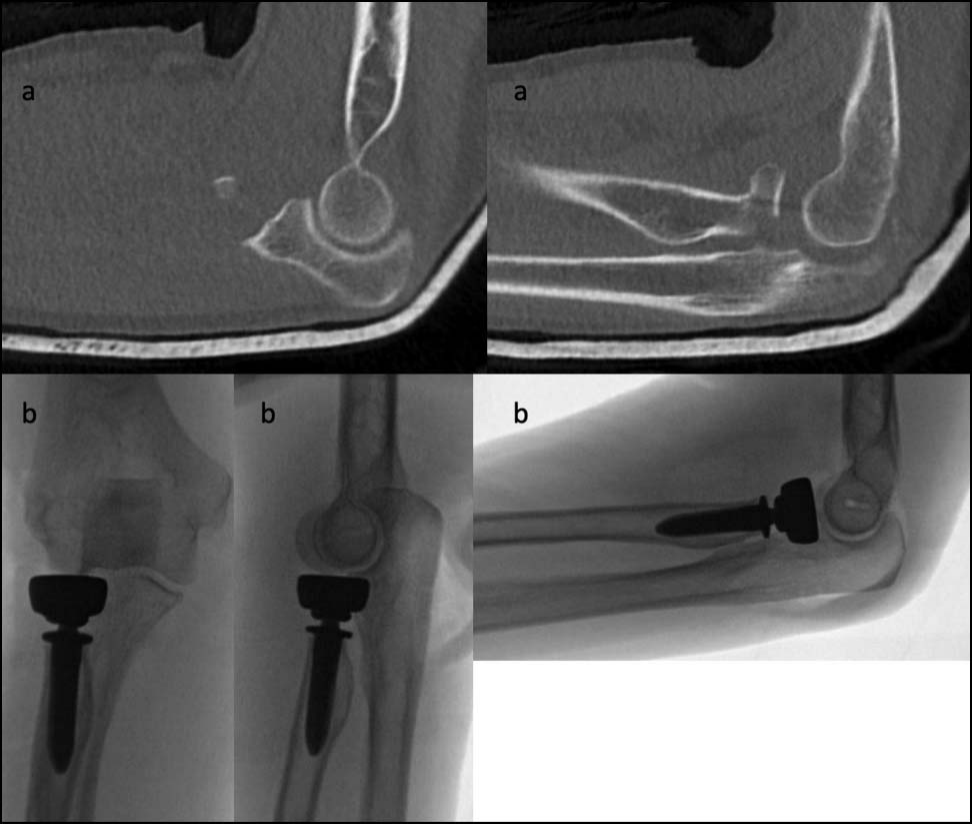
Postreduction CT scans demonstrating terrible triad injury of the elbow with radial head and coronoid fracture (A). Radiographs demonstrating surgical fixation using a radial head prosthesis (B).
